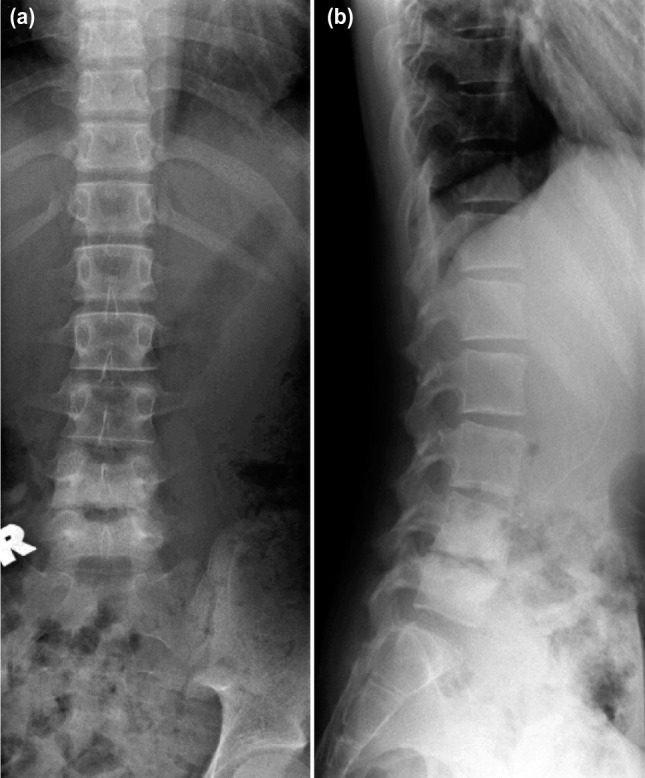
(a) Radiograph of lumbosacral anteroposterior view showing reduced joint space with end plate erosion over L4-L5 region and (b) Radiograph of lumbosacral lateral view showing reduced joint space, end plate erosion over L4-L5 vertebra and also loss of lumbar lordosis.
